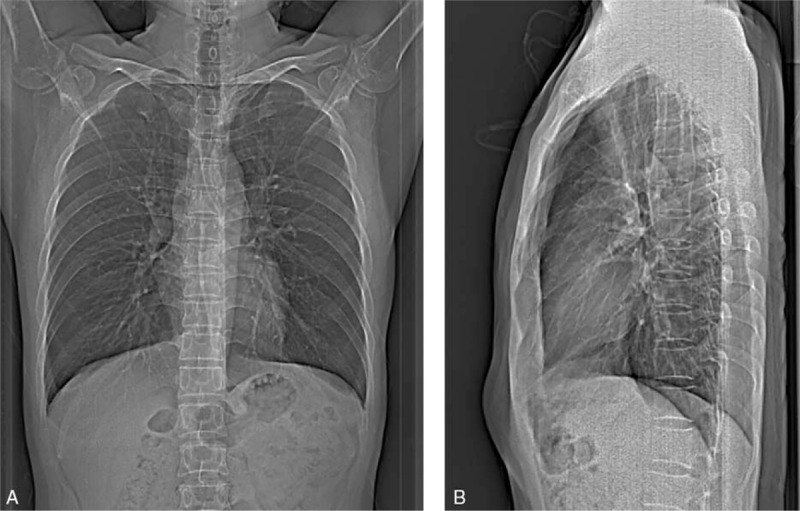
An example of the AP (A) and lateral (B) scout views used for chest CT scanning. AP = anteroposterior, CT = computed tomography.
